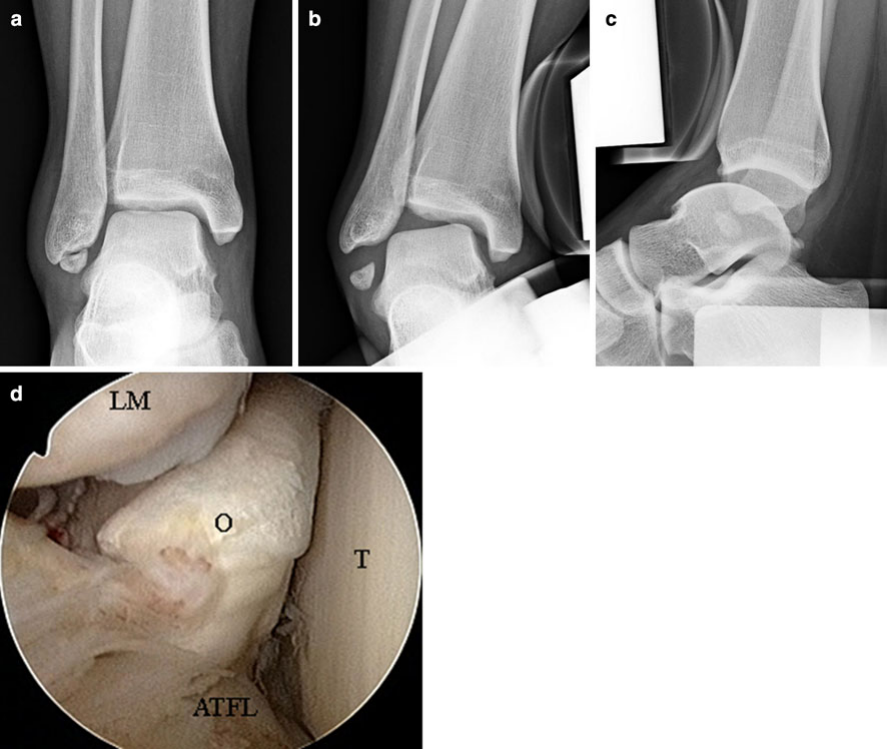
Case showing no indication for arthroscopic excision (15-year-old female). Anteroposterior radiograph (a) showing a large ossicle. Varus stress radiograph (b) and anterior stress radiograph (c) showing high instability of the ankle and an opening between the ossicle and lateral malleolus. Arthroscopic findings (d) showing a large gap between the ossicle and lateral malleolus (*O* ossicle, *T* talus, *ATFL* anterior talofibular ligament, *LM* lateral malleolus)
